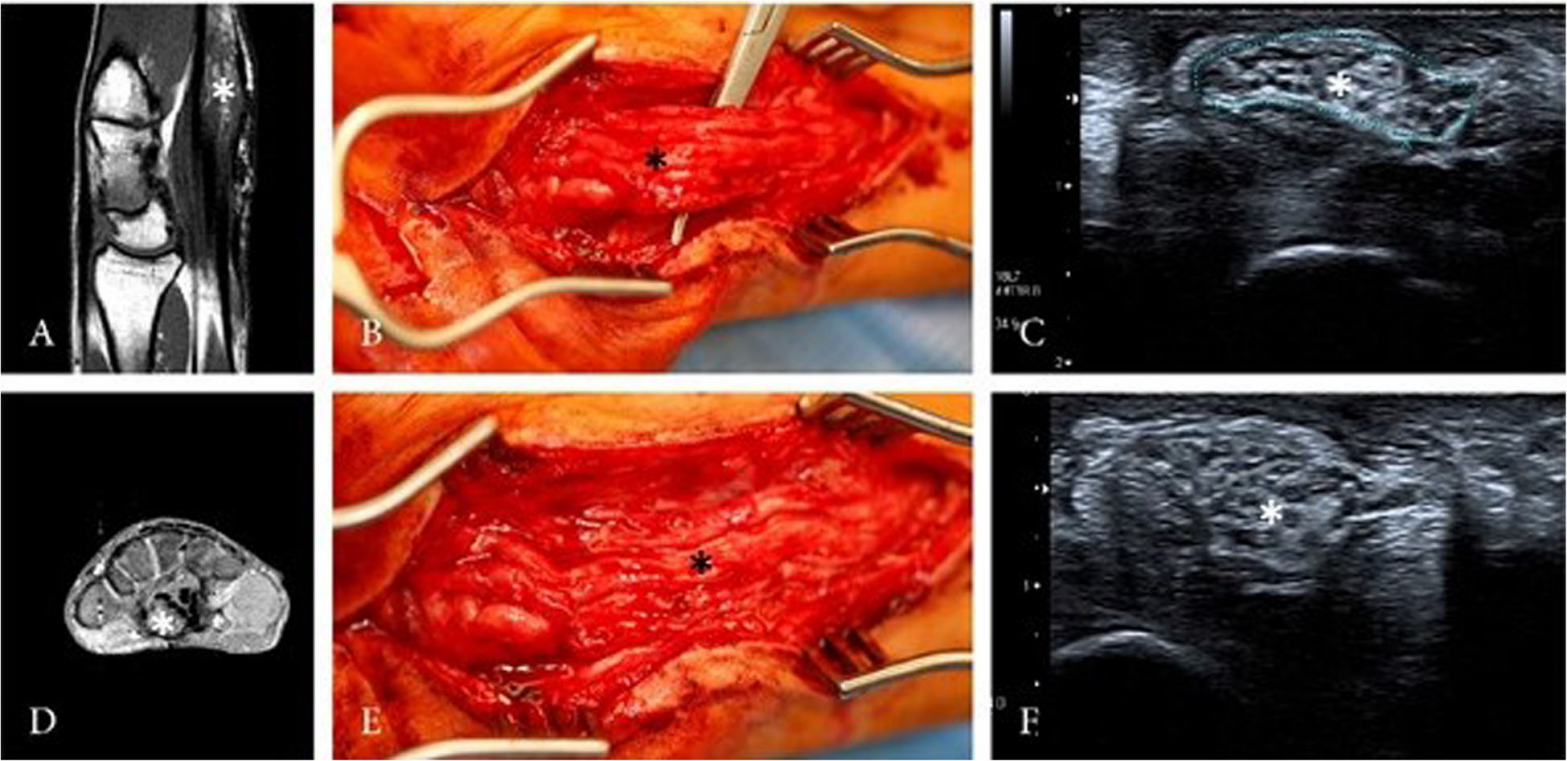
HRNS (c + f) and MRI (a + d) of the right-hand depicted nerve enlargement (asterisk) with perineurial tissue and still identifiable fascicular structure. Intraoperative view showing the perineurioma (b + e)
